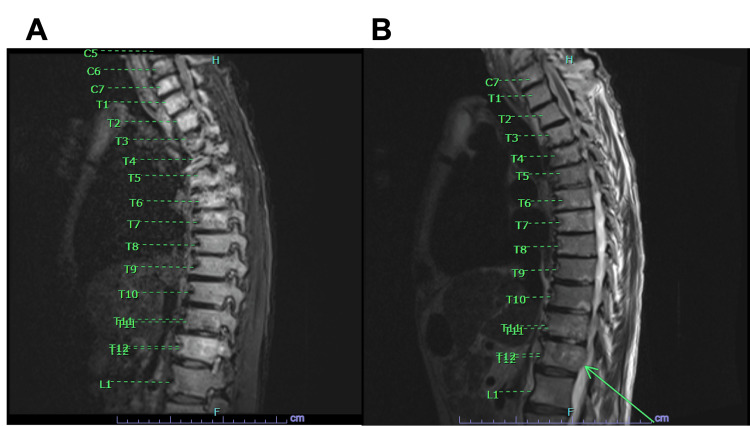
(A) A STIR MRI sequence of the lower cervical and thoracic spine. The radiologist noted diffuse marrow heterogeneity specifically in C7, T5, and T12 vertebral bodies. (B) A T2 weighted MRI demonstrating a sliver of epidural soft tissue along the right aspect of T12, noted with an arrow. No significant cord compression was observed, however. STIR: Short tau inversion recovery
